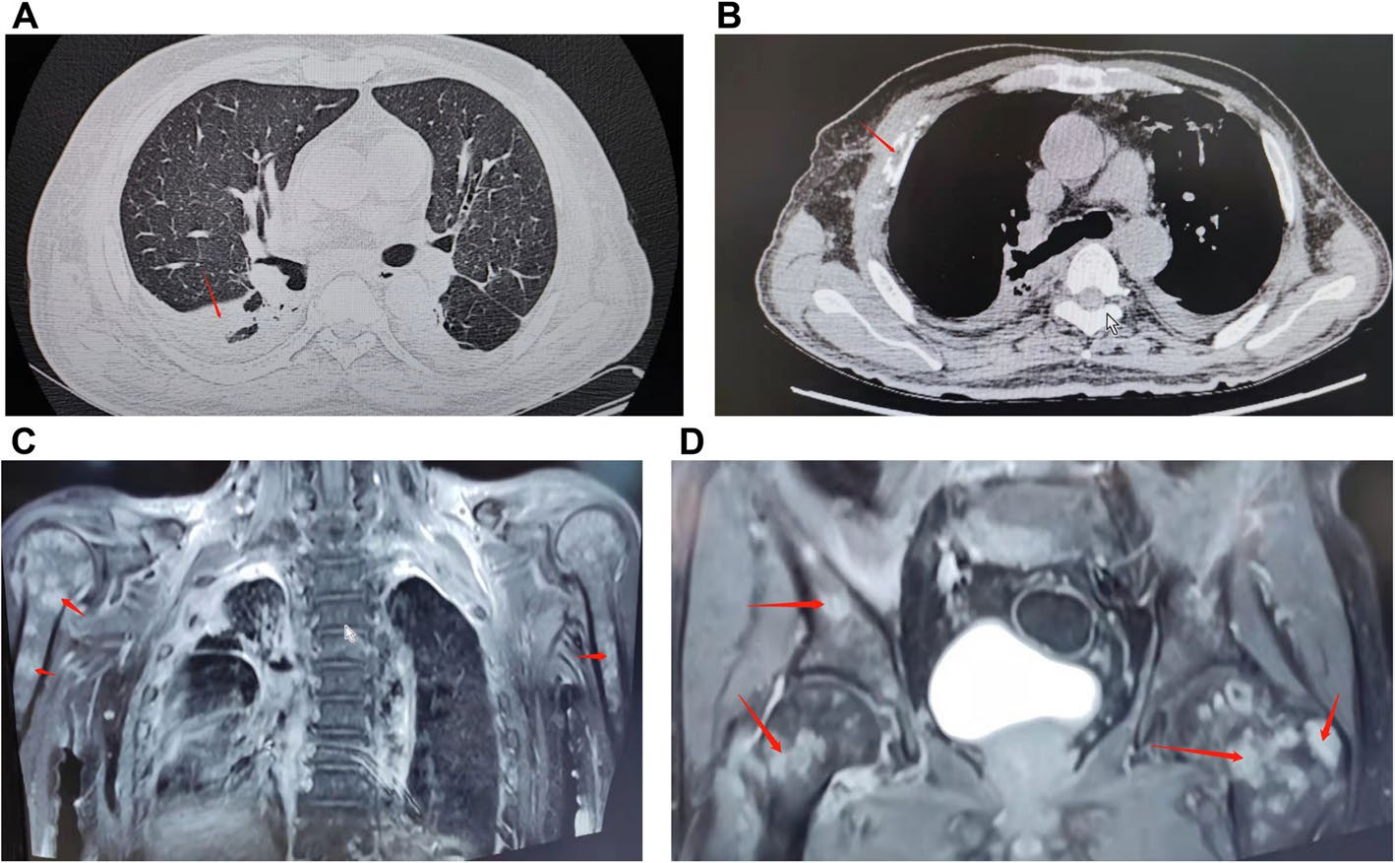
A–B Chest CT image showing alveolar consolidation in the left upper lobe (arrows) and chest wall rib destruction (March 2019) (arrows). C–E Spinal and pelvic MRI images showing bone destruction in the spine, sternum, scapula, humerus, and pelvis (arrows). CT, computed tomography; MRI, magnetic resonance imaging;
